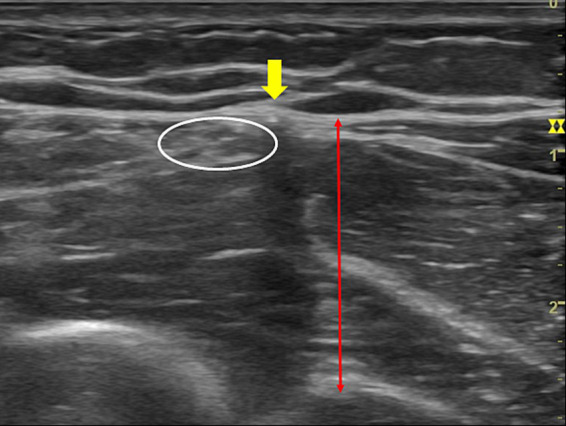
High-resolution ultrasound image of the non-palpable etonogestrel implant (yellow arrow) abutting the ulnar nerve sheath (white circle) in a 33-year-old woman (Busan, South Korea, 2025); bilateral red arrows indicate posterior acoustic shadowing beneath the etonogestrel implant. UN, ulnar nerve; US, ultrasound.
